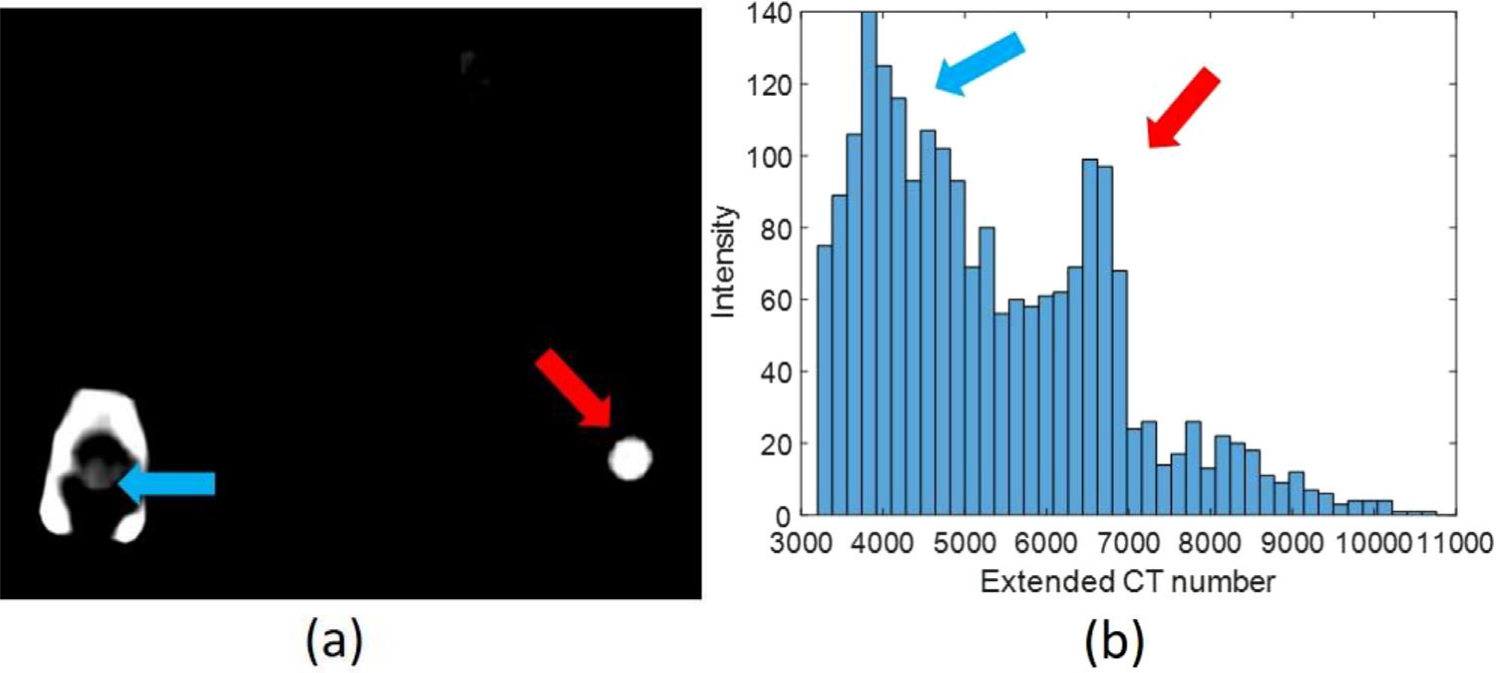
(a) A patient computed tomography (CT) image with extended CT scale, a 125‐mm‐reconstruction diameter, and adjusted window level to emphasize the implant position; (b) the histogram of extended CT scale for all spine screw system in a patient
